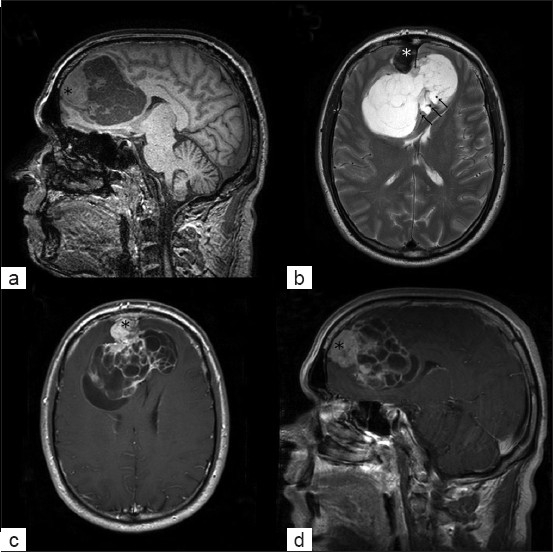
Magnetic resonance imaging of a large frontal interhemispheric heterogeneous lesion; (a) sagittal T1-weighted scan; (b) axial and coronal T2-weighted images showing the important mass-effect and the partial inclusion of both anterior cerebral arteries and their branches in the tumor (arrows); (c) and (d) axial and sagittal gadolinium-enhanced T1-weighted images showing both the anterior solid (asterisk) and the multicystic components of the lesion with the homogenous enhancement of the solid part, as well as the enhancement of the thick cystic walls
